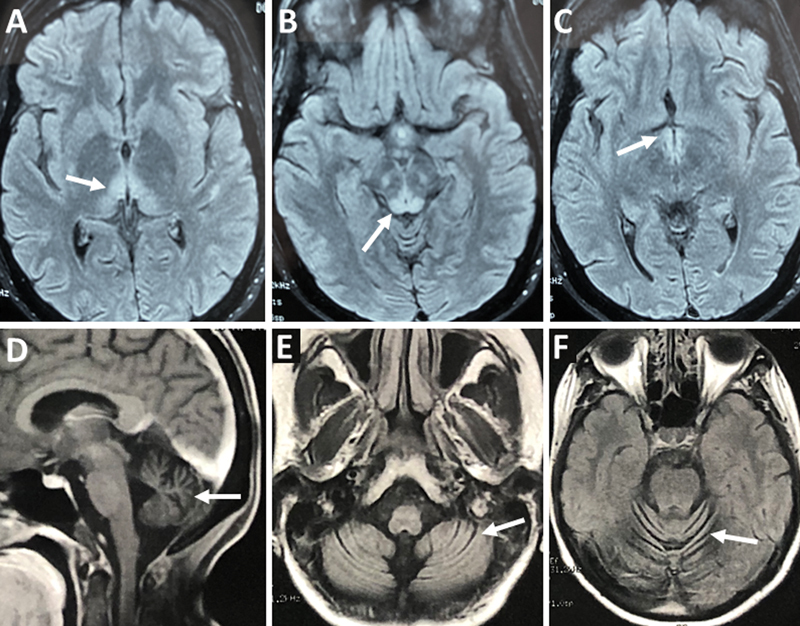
Patient 1: axial fluid-attenuated inversion recovery (FLAIR)-weighted brain magnetic resonance imaging (MRI) scan showing bilateral hyperintense signal in the medial thalami (
